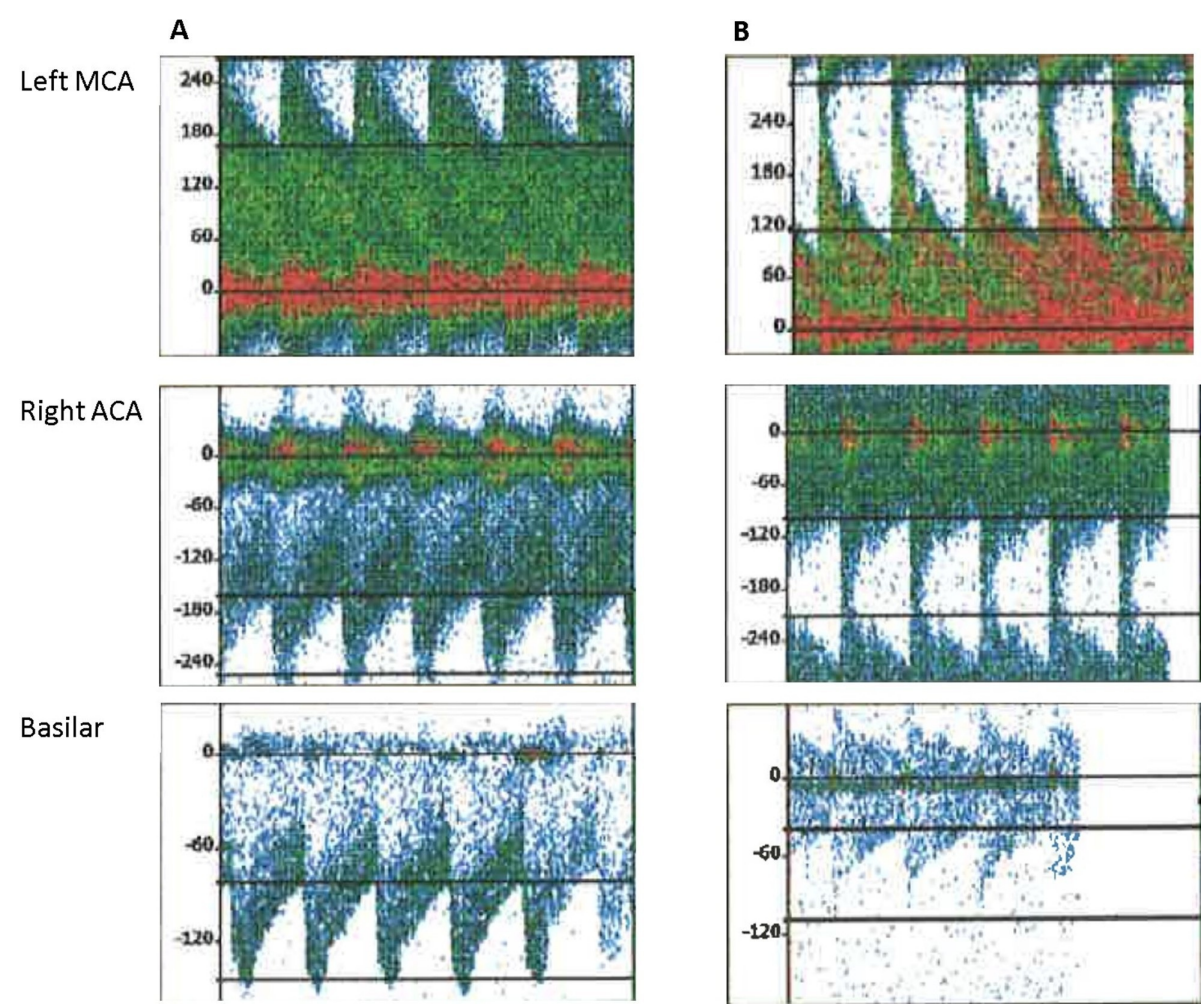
Transcranial Doppler (TCD) waveforms Transcranial Doppler (TCD) waveforms from the left middle cerebral artery, right anterior cerebral artery, and basilar artery, showing the pre-milrinone waveforms (A) and the post-milrinone waveforms (B).
